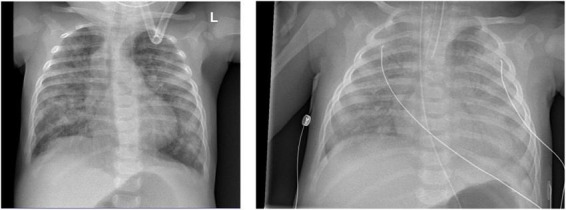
Chest X-ray of patient six at 4 months of age shows lung infiltrations and development of acute respiratory distress syndrome (ARDS, pneumocystis carinii and streptococcus pneumonia).
